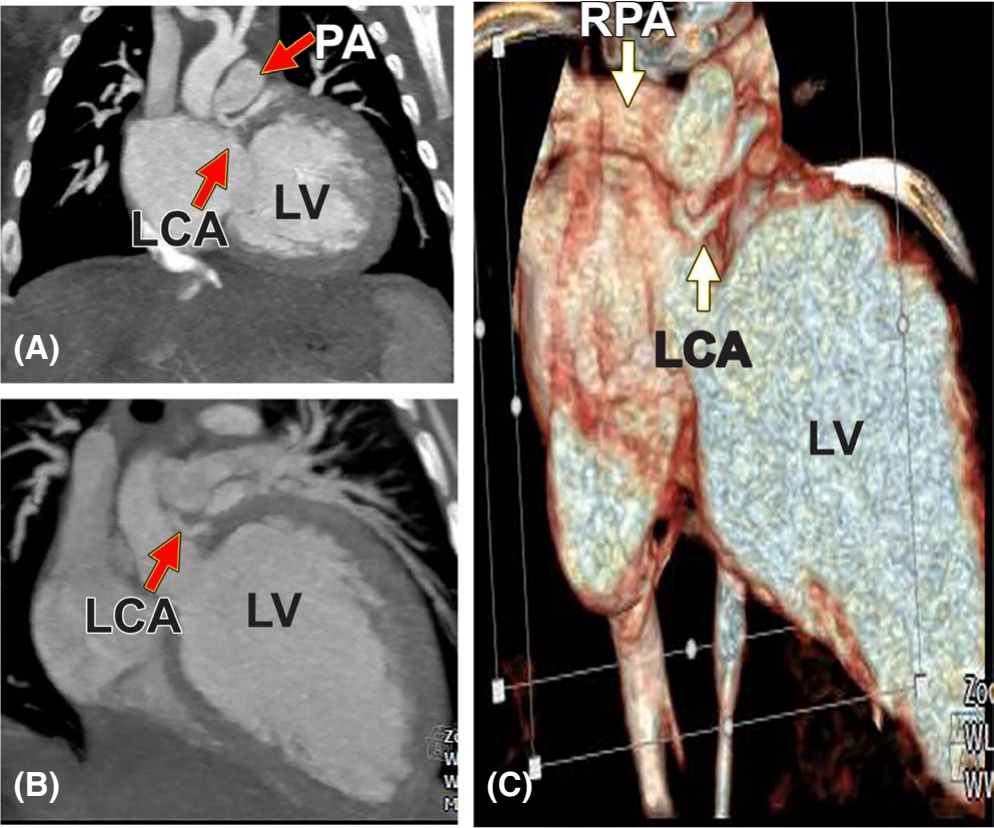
384‐slice coronary computed tomography angiography. A and B show the origin of the anomalous left coronary artery. C, this volume‐rendered image indicates that the orifice of the anomalous left coronary artery is in the proximal right pulmonary artery. PA = pulmonary artery, RPA = right pulmonary artery, LV = left ventricle, LCA = left coronary artery
